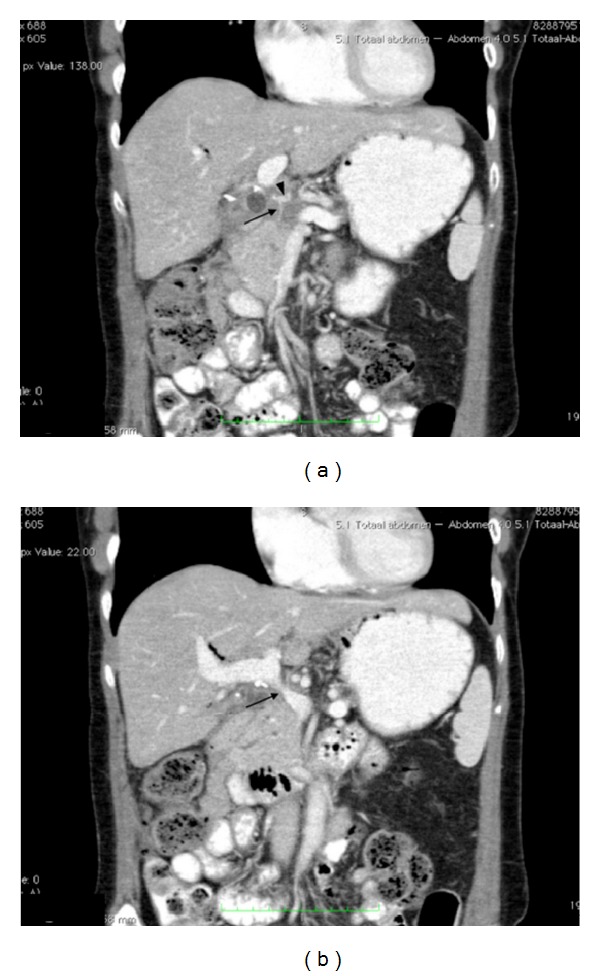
(a) 3 months interval portal venous-phase abdominal CT scan (coronal view). Status after exploratory laparotomy and 6 courses of chemotherapy. Note hypodensity in pancreatic head with encasement of the gastroduodenal artery (arrow) and common hepatic artery (arrowhead). (b) 3 months interval portal venous-phase abdominal CT scan (coronal view). Status after exploratory laparotomy and 6 courses of chemotherapy. Progressive stenosis of the portal vein can be noted (arrow).
